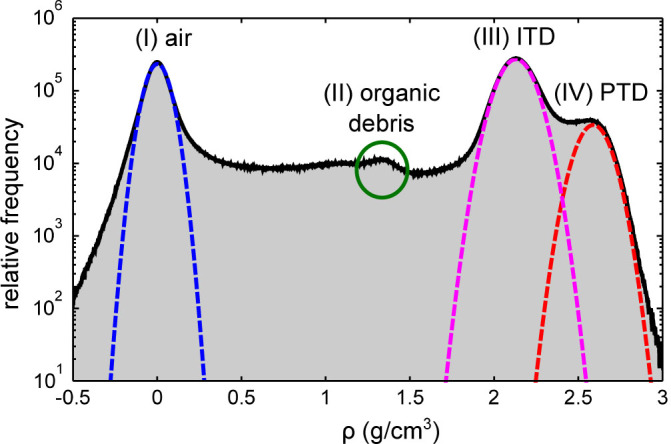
Histogram of dentine obtained by ptychographic X-ray nanoCT. The four peaks (I) to (IV) correspond to air and the three materials typically found in dentine. Gaussian fits to the air (I), intertubular dentine ITD (III) and peritubular dentine PTD (IV) peaks are shown with dashed color lines.
